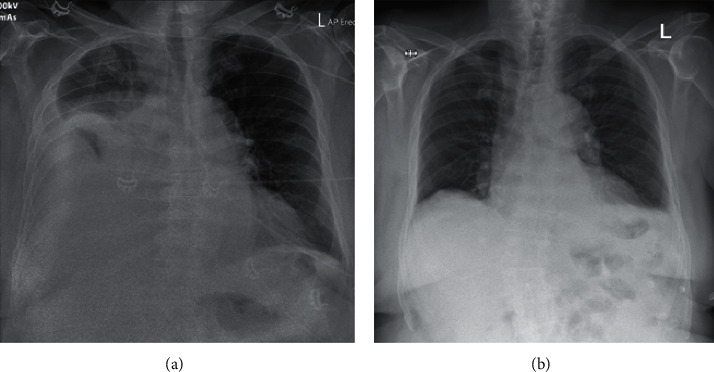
Chest X-ray on presentation (a) and after thoracentesis (b).
